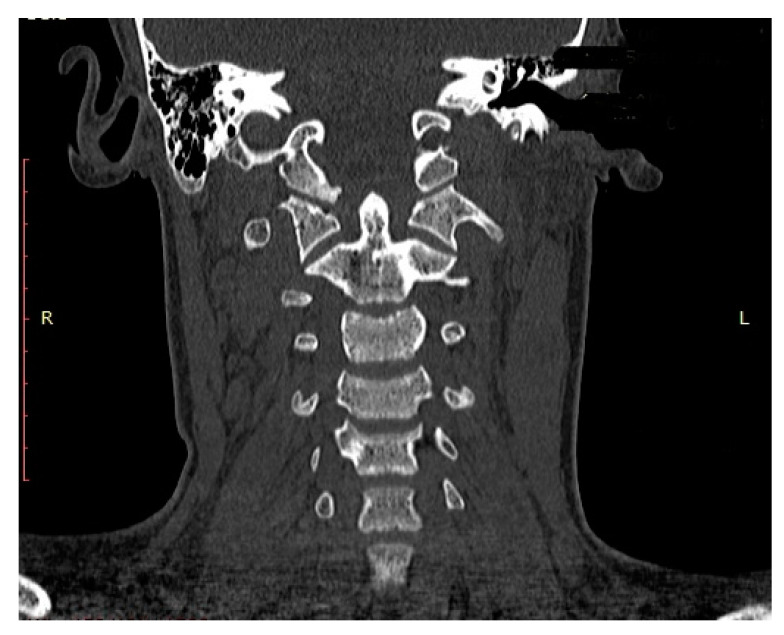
CT scan (frontal plane) of a boy aged 15.2 years (patient: P.P.) obtained after halo-vest therapy, showing bony consolidation of right-sided OCF in correct alignment.
